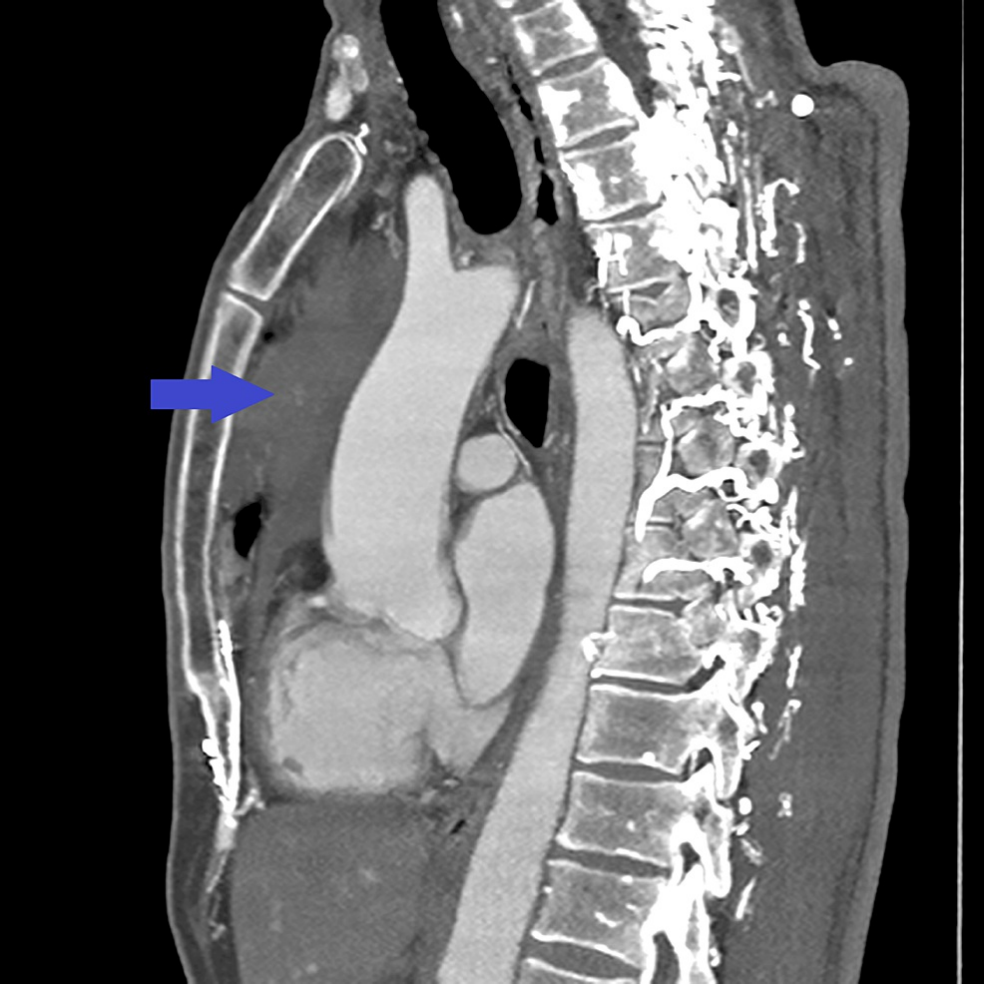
Enhanced CT scan of the chest showing a large anterior mediastinal mass (blue arrow).
